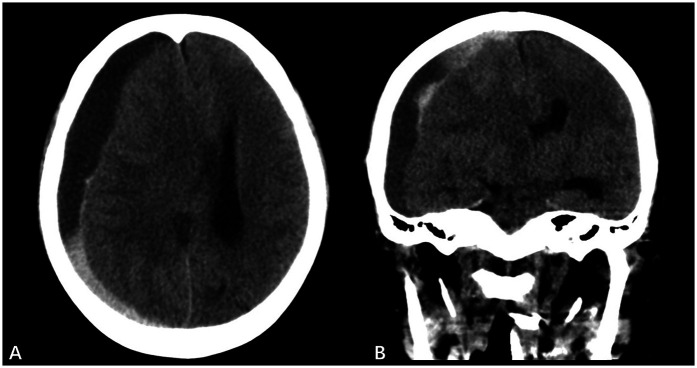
Preoperative CT scans (A) and (B) demonstrate: right fronto-temporo-parieto-occipital subdural hematoma, brain herniation, Minor subarachnoid hemorrhage, midline shift.
